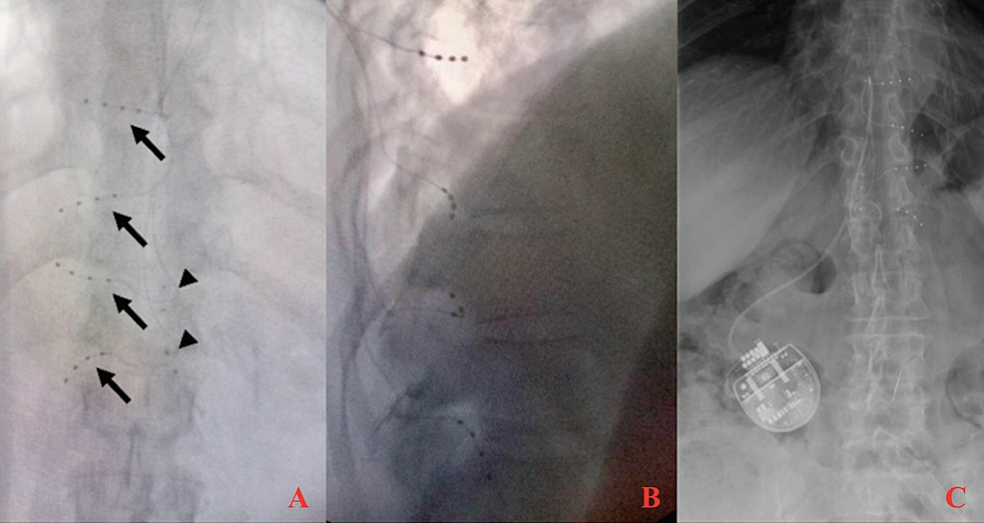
Anteroposterior (A) and lateral (B) fluoroscopic views demonstrating DRG electrodes (black arrows) exiting the left T9-T12 intervertebral foramina with titanium plates (arrowheads) anchoring the T11 and T12 electrodes. Additionally, a six-month follow-up abdominal x-ray (C) demonstrating a stable position of the DRG electrodes.
